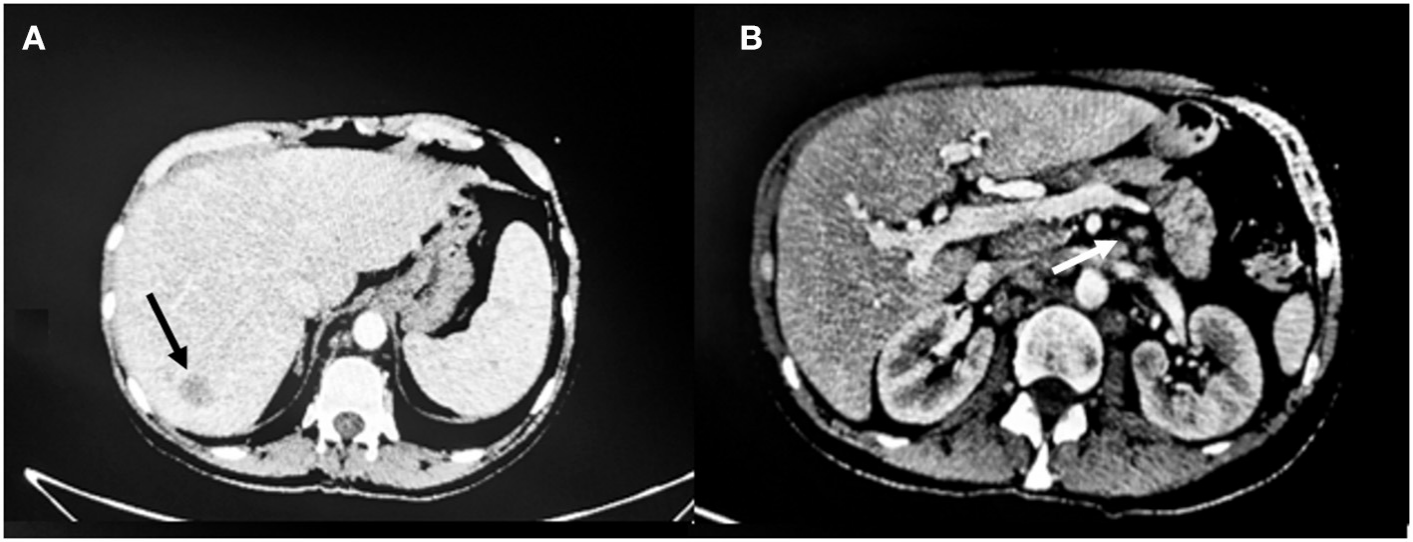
CT scan findings 20 days post-operatively. (A) The arrow shows one of the three hypointense hepatic lesions and periportal halo. (B) Dilatation of the intrahepatic biliary tree and inhomogeneous enhancement of the hepatic parenchyma as for cholangitis with increase in size and number of the splenic hilar (arrow), hepatic hilar and paraaortic lymph nodes.
